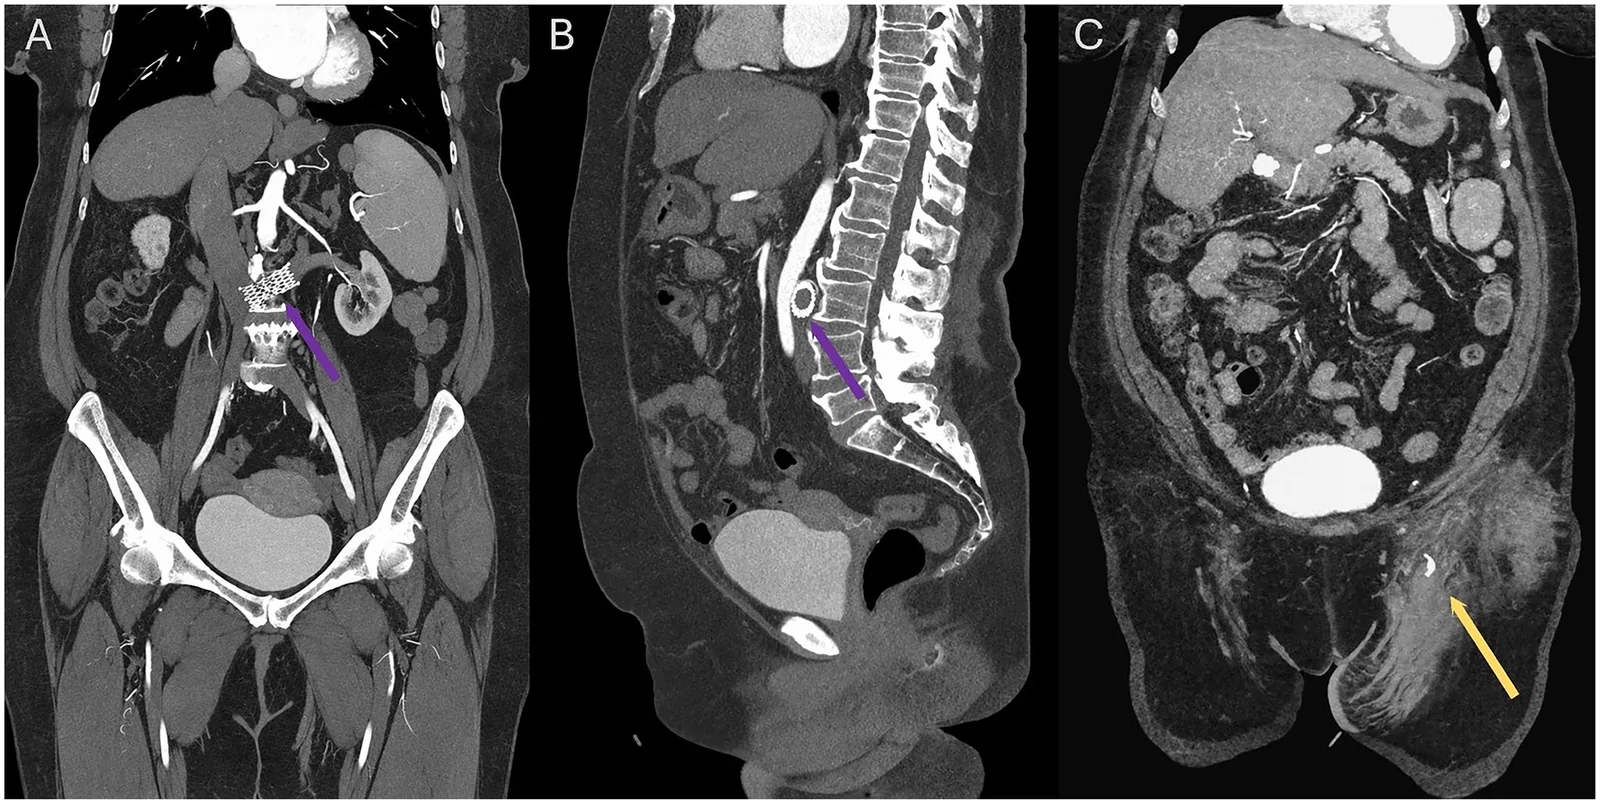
Postprocedural contrast-enhanced CT scan. (A) and (B) Patent left renal vein stent (purple arrows). (C) Active bleeding from the left femoral vein access site with associated proximal thigh haematoma (yellow arrow).
